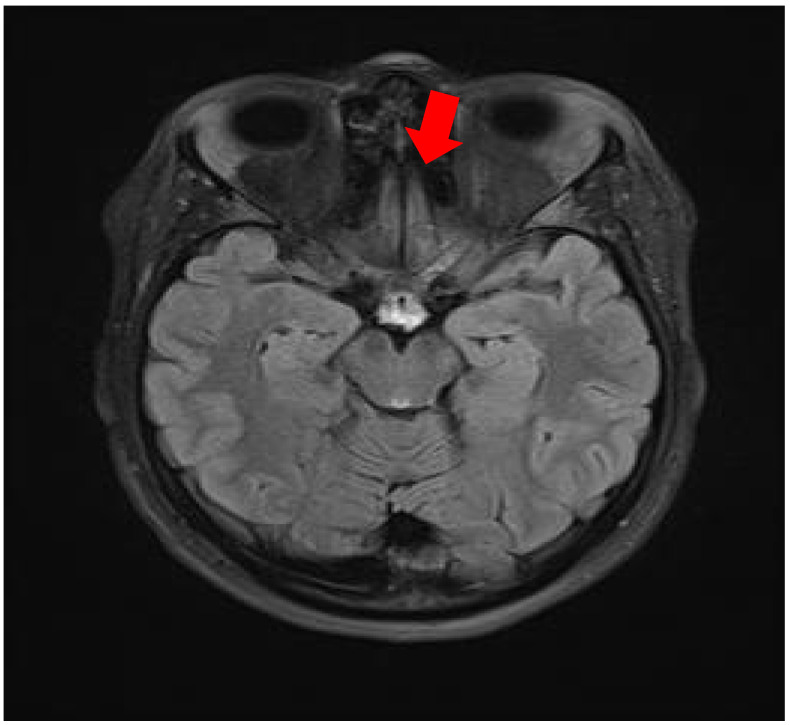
Paranasal sinus MRI: Mucosal thickening in the anterior nasal cavities with partial obstruction of the maxillary ostia (arrow), suggestive of inflammatory/granulomatous involvement.
